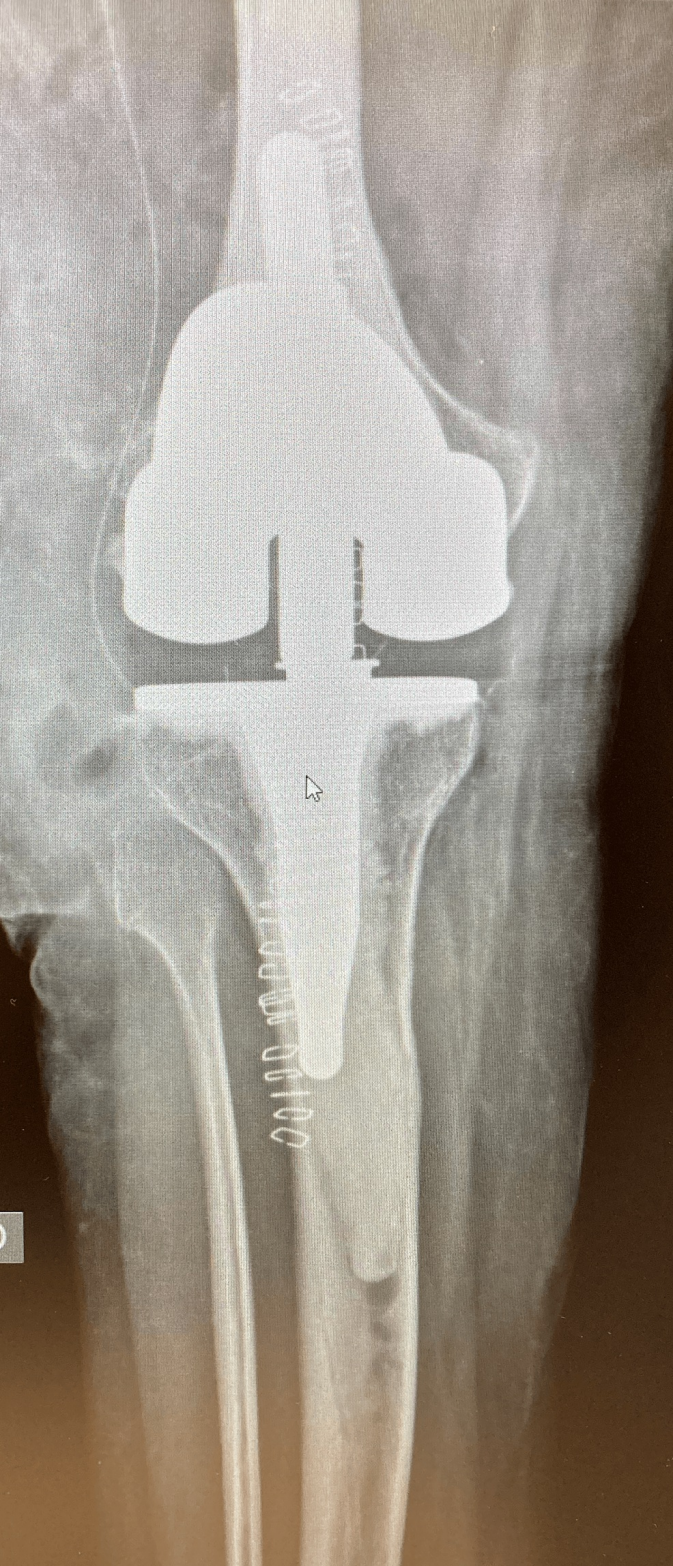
Postoperative anteroposterior knee radiograph showing the cemented rotating-hinged total knee arthroplasty.
